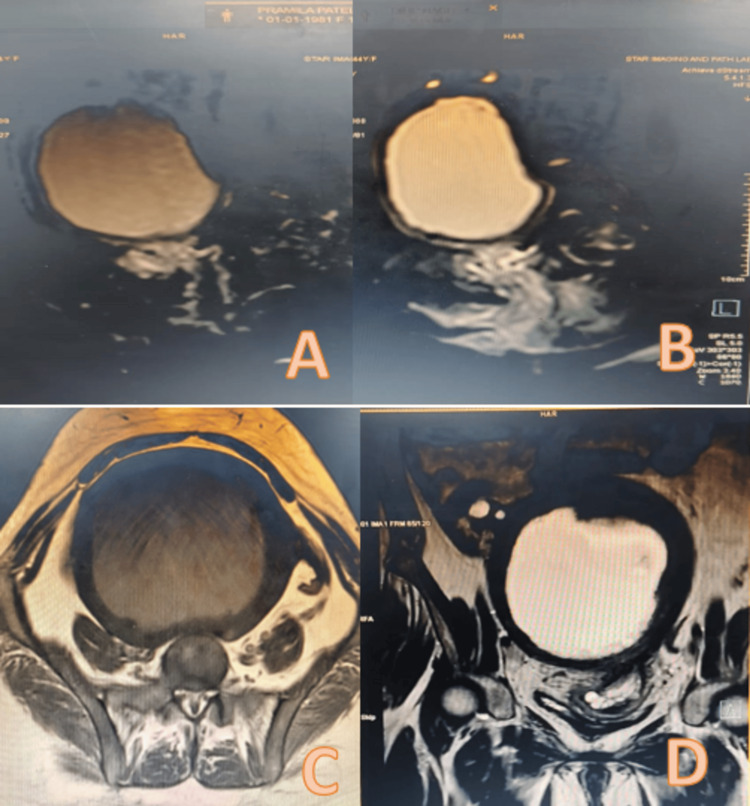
Diffusion-weighted MRI of the pelvic mass (A) Diffusion-weighted image (DWI, b=1000 s/mm²) demonstrating marked hyperintensity within the large pelvic mass. (B) Corresponding apparent diffusion coefficient (ADC) map showing low ADC signal (diffusion restriction) in the same region, raising suspicion of a malignant uterine neoplasm. (C) Axial T1-weighted MRI demonstrating a large heterogeneous pelvic mass with predominant T1 hyperintensity, suggestive of hemorrhagic/proteinaceous content. The marked T1 hyperintensity, together with diffusion restriction on DWI/ADC sequences, contributed to the preoperative radiologic suspicion of malignancy. (D) Coronal T2-weighted MRI of the pelvis demonstrates a markedly distended fluid-filled uterine cavity with homogeneous T2 hyperintense contents. A well-defined hypointense soft-tissue lesion is observed arising from the lower uterine segment/cervical region, causing obstruction of the endometrial cavity. No obvious large complex adnexal mass is identified on this image.
